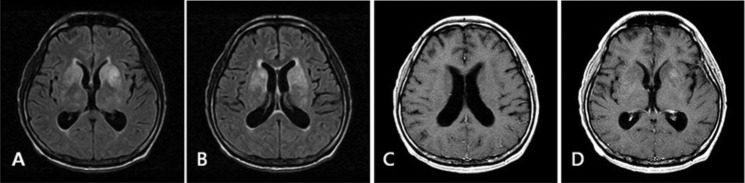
Axial FLAIR (A and B) and T1WI postcontrast (C and D) imaging show hyperintense signal in both the basal ganglia and thalamus with focal enhancement on the left putamen.
